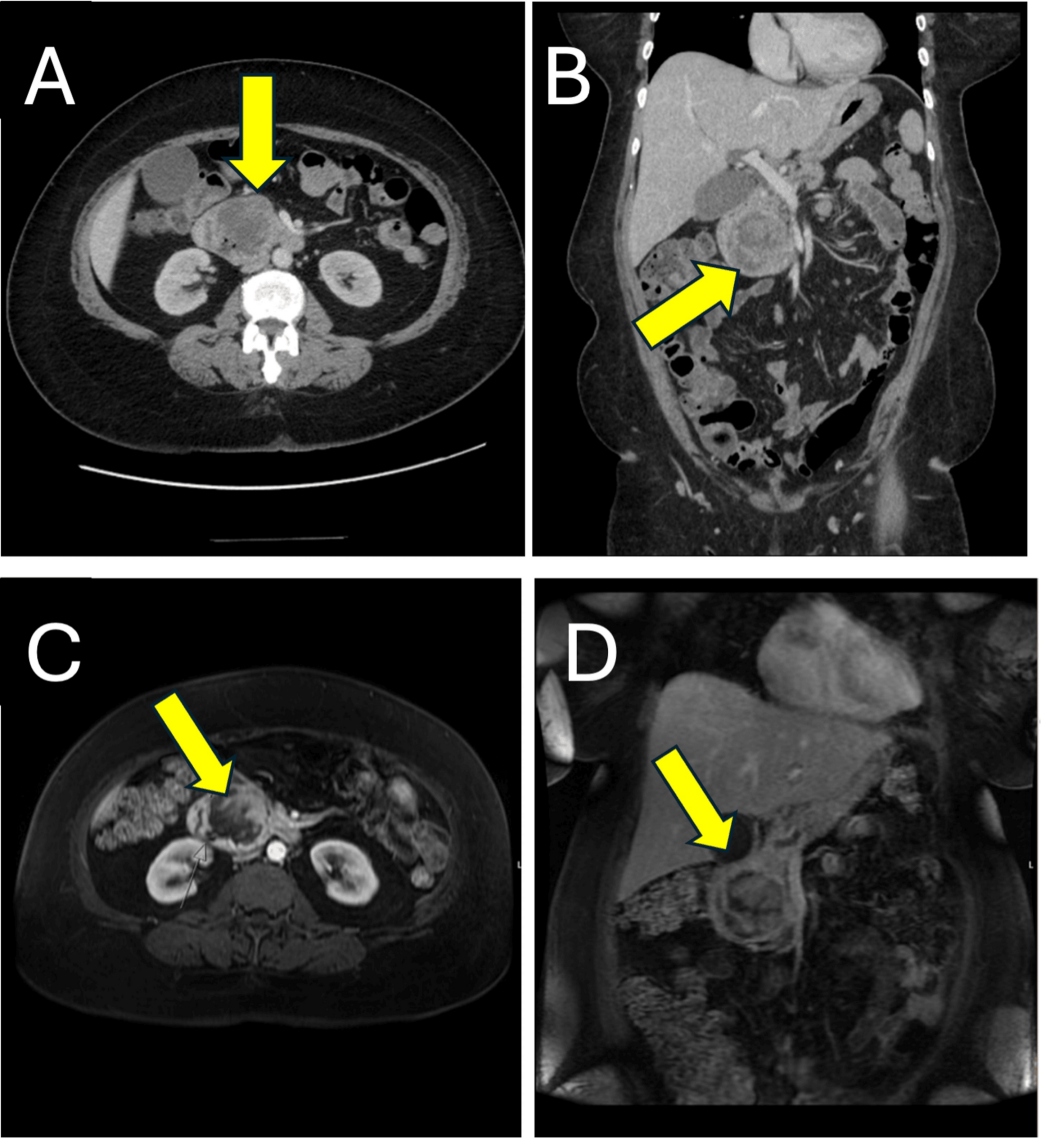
CT of the chest, abdomen, and pelvis with contrast and MRI of the primary pancreaticoduodenal soft tissue mass. (A) CT axial view, (B) CT coronal view, (C) MRI axial view, (D) MRI coronal view, with the yellow arrow pointing to an expansile mass (5.4×5.1 cm) in the region of the duodenum and pancreatic head, with no evidence of metastatic disease. CT, computed tomography; MRI, magnetic resonance imaging
